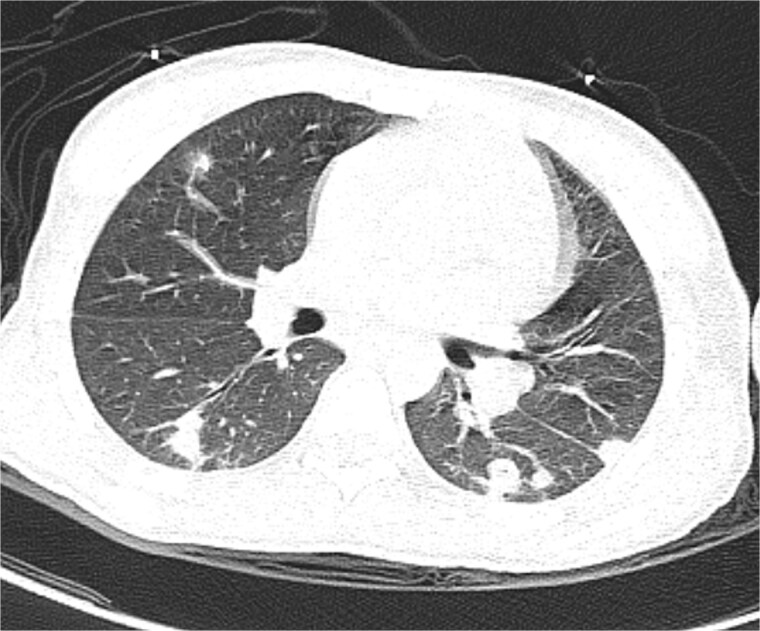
Axial chest CT (parenchymal window) demonstrating septic emboli.
